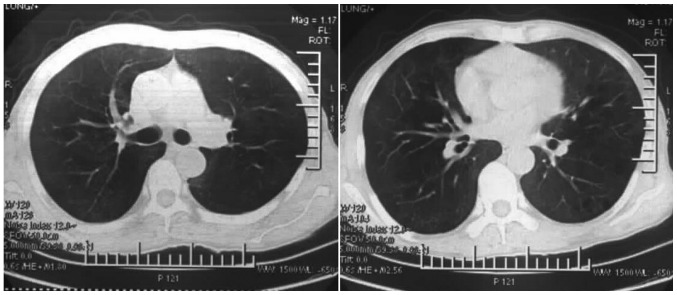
Chest computed tomography on October 15, 2007: Normal lungs.
